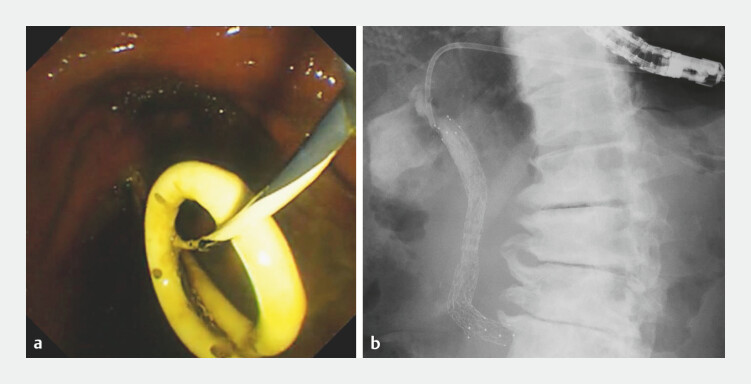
Endoscopic reintervention was performed 16 days after the initial endoscopic ultrasound-guided hepaticogastrostomy (EUS-HGS).
